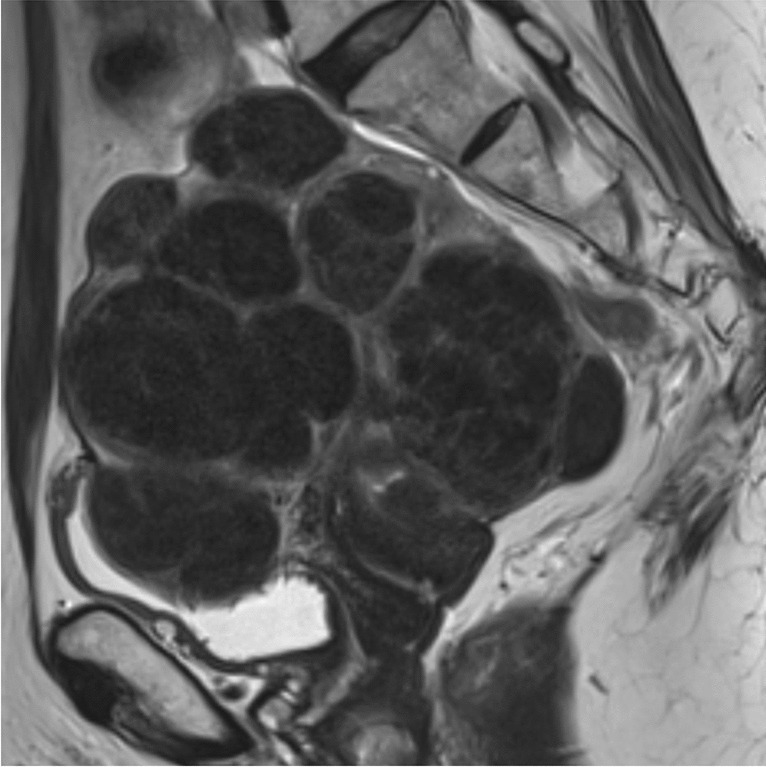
T2-weighted sagittal image of the pelvis demonstrating multiple large T2 hypointense fibroids which occupy most of the pelvic cavity and are associated with significant mass effect on surrounding pelvic structures
